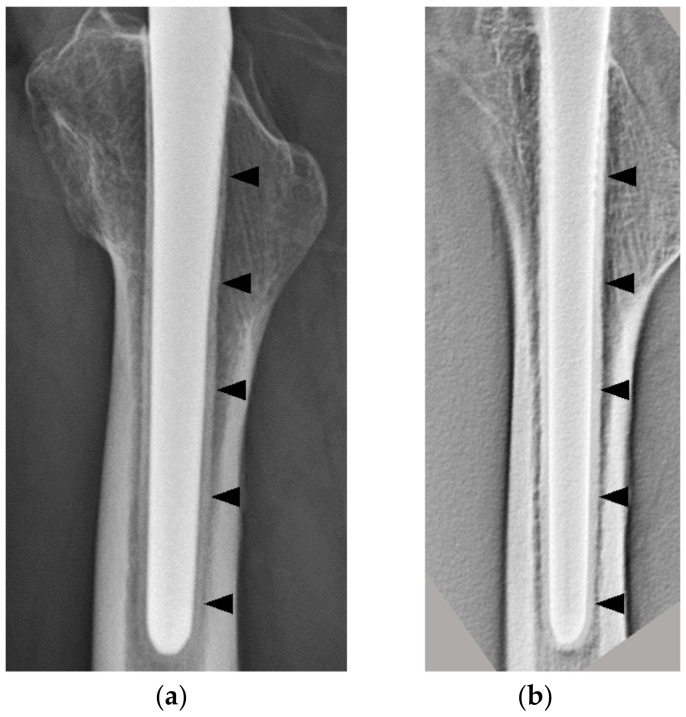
An 81-year-old female patient 3 y after THA. (a) Plain radiographs (lateral view); there was bone formation surrounding the stem (black arrowheads). (b) DTS (lateral view); in the same patient as in (a), bone formation was observed uniformly around the stem from proximal to distal (black arrowheads), and the stem–bone interface is seen more clearly in (b) than in (a). THA, total hip arthroplasty; DTS, digital tomosynthesis.
